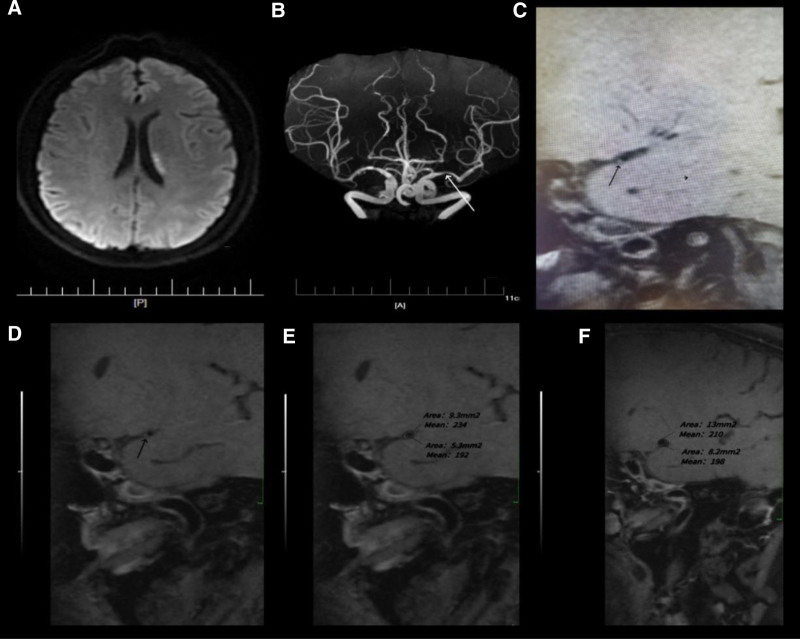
HR-MRI of the symptomatic middle cerebral artery (MCA) stenosis in a 67-yr-old female presenting with right upper limb weakness lasting 16 h. (A) Diffusion weighted imaging (DWI) reveals an acute ischemic infarct in the left MCA territory. (B) TOF-MRA demonstrates severe stenosis in the M1 segment of the left MCA (white arrow). (C) T1-weighted HR-MRI identifies the plaque (black arrow). (D) Post-contrast T1-weighted HR-MRI shows eccentric wall thickening without evident enhancement at the plaque site (black arrow). (E) At the point of maximal narrowing, post-contrast T1-weighted HR-MRI shows a V of 9.3 mm^2^ and an LA of 5.3 mm^2^. (F) The vessel area is 13.0 mm^2^ at the reference site, and the LA is 8.2 mm^2^. The degree of stenosis is calculated as: (1–5.3 mm^2^/ 8.2 mm^2^) × 100% = 35.37%. HR-MRI = high-resolution magnetic resonance imaging, LA = lumen area, TOF-MRA = time-of-flight magnetic resonance angiography.
